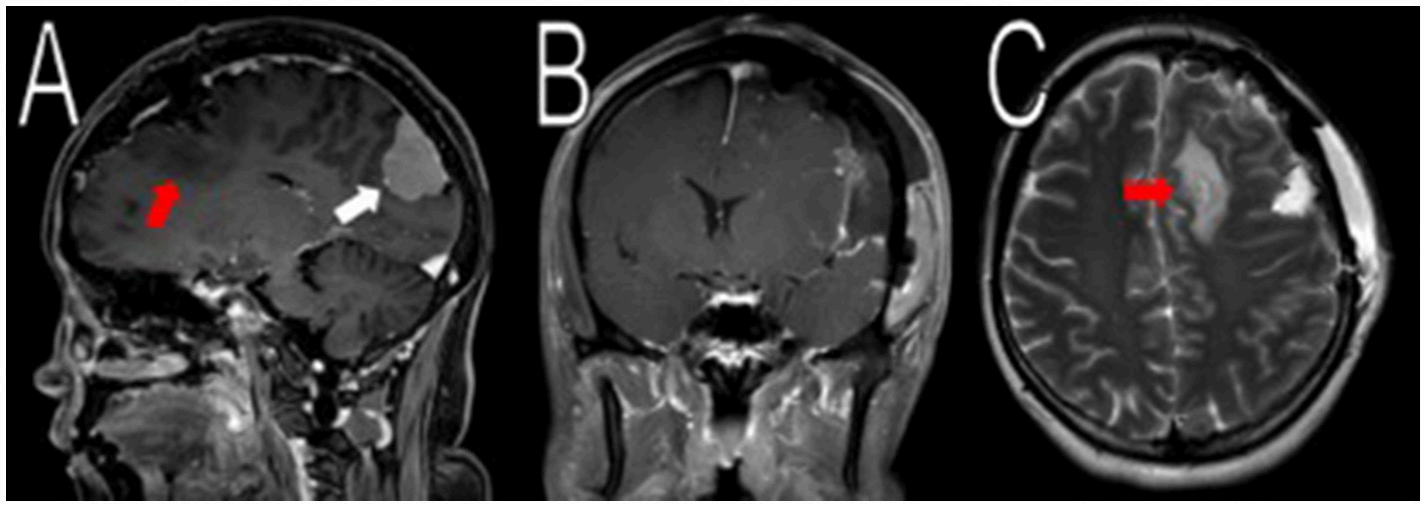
Resection of the frontal/parietal/temporal mass. Post-operative MRI contrasted T1 and T2 scans showed resection of the meningiomas in the left frontal/parietal/temporal convexity with expected post-operative changes (red arrows in A,C, not shown in B). The occipital lobe mass was visible from the sagittal view (white arrow in A).
